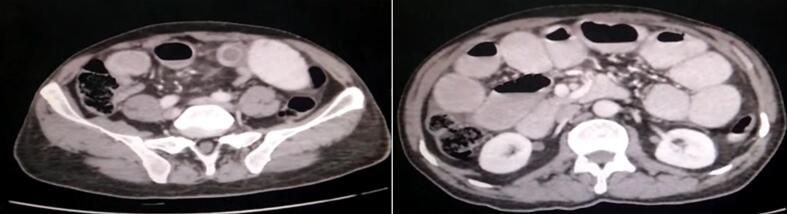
Axial CT abdomen with contrast showing a large stone impacted in the distal ileum with proximal small bowel dilatation and distal collapse.
